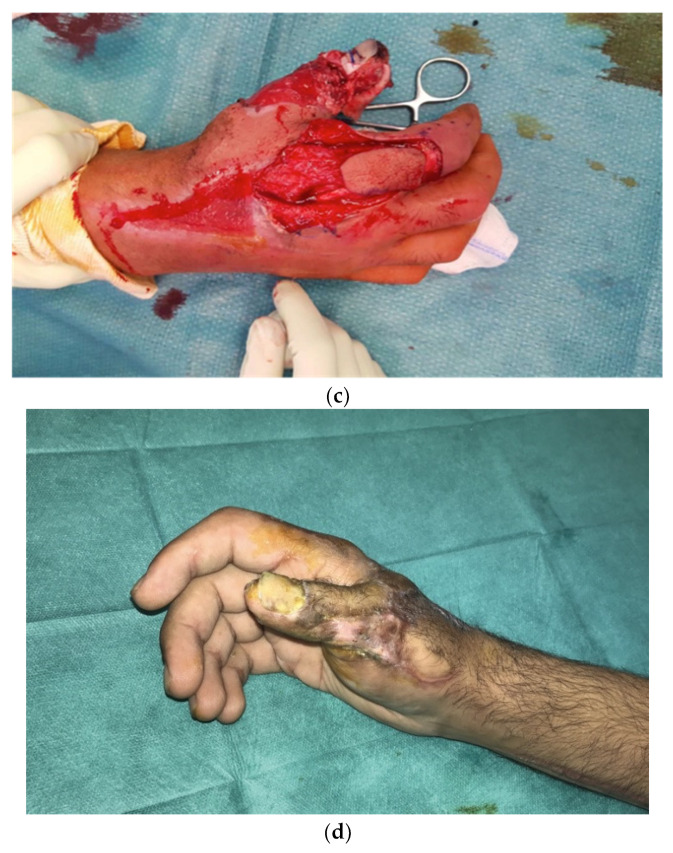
A 27-year-old patient presented with the clinical signs of necrotizing fasciitis of the right hand following an injury involving a wrought-iron handle 48 h earlier (a). Exploration of the vascular pedicles of the right thumb had revealed contracted vessels surrounded by infected tissue; a precautionary debridement was performed to preserve the vascular pedicles (b). The necrotic areas and all the non-viable tissue of the right thumb were debrided, with excision of the long extensor tendon of the thumb. The IP joint was opened, revealing the presence of pus; the cartilage was then resected until healthy, vascularized bone was reached. Following liberal irrigation of the articulation and tissues using Betadine^®^, we ensured arthrodesis of the thumb IP joint with two pins using X-ray guidance. To cover the loss of soft tissue on the dorsal side of the thumb, leaving the IP joint exposed, it was decided to perform a kite-shaped dorsal metacarpal artery flap. The flap was drawn on the dorsal side of the first phalange of the second finger, going from the extension fold of the PIP, up to the metacarpophalangeal joint and laterally to the mediolateral line (c). The flap donor site and the flap pedicle were covered by a full-thickness skin graft removed from the inner surface of the right arm. Six months later, the result was satisfactory, with good functional recovery and articular mobility (d).
